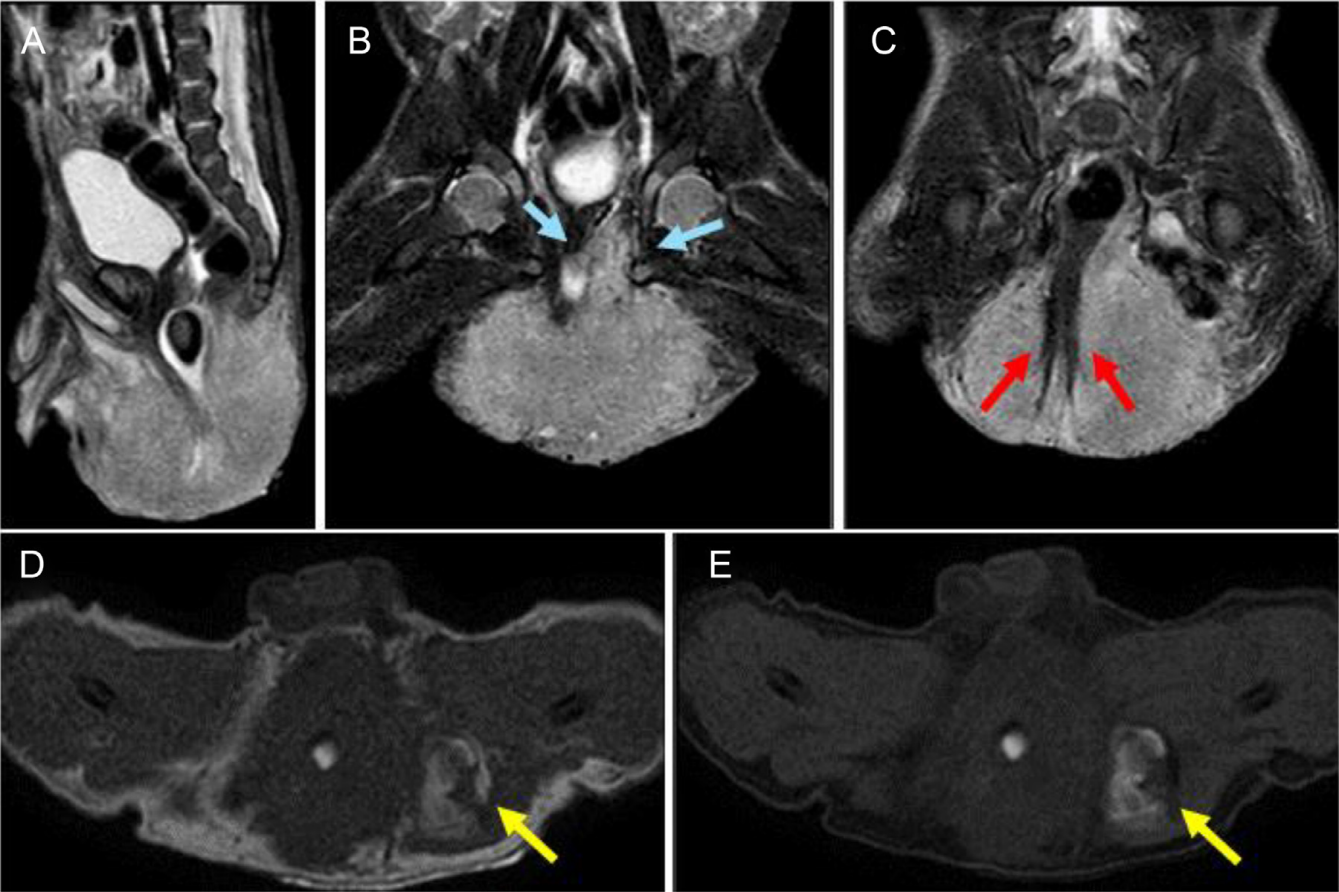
Postnatal MRI performed at 3 days old. Sagittal STIR (A) shows the pelvic mass exophytic to but not invading the spine. Coronal STIR imaging (B, C) shows tumor extension through the left inguinal canal (blue arrows). The mass displaces the rectum and anus which has a tethered appearance (red arrow). Axial T1 weighted image without (D) and with (E) fat saturation show hyperintense signal (yellow arrow) within the mass, which does not lose signal with fat saturation (yellow arrow), compatible with hemorrhage.
